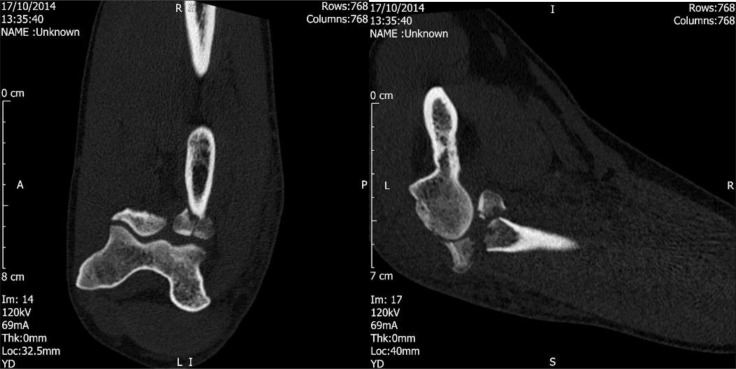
Pre-operative CT images of type III injury.
